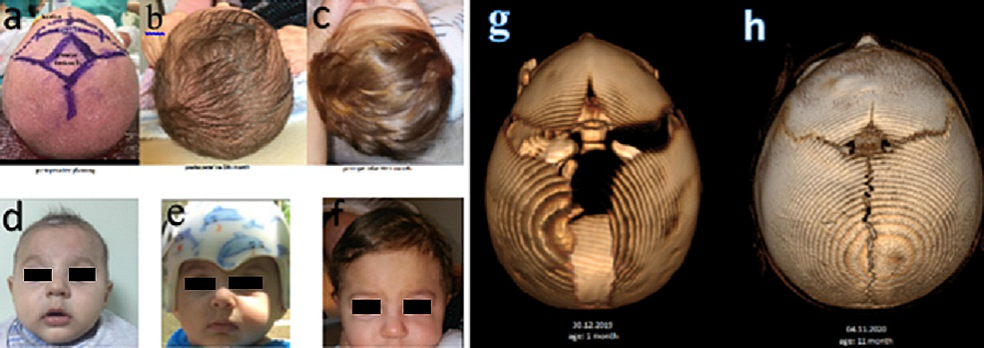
Endoscopy-assisted suturectomy Preoperative preparation (a), postop second-month (d), postop sixth-month (b,e) (helmet) and 11th-month (c,f) clinical appearance. Preop (g) and postop 11th-month (h) 3D CT CT: computed tomography
